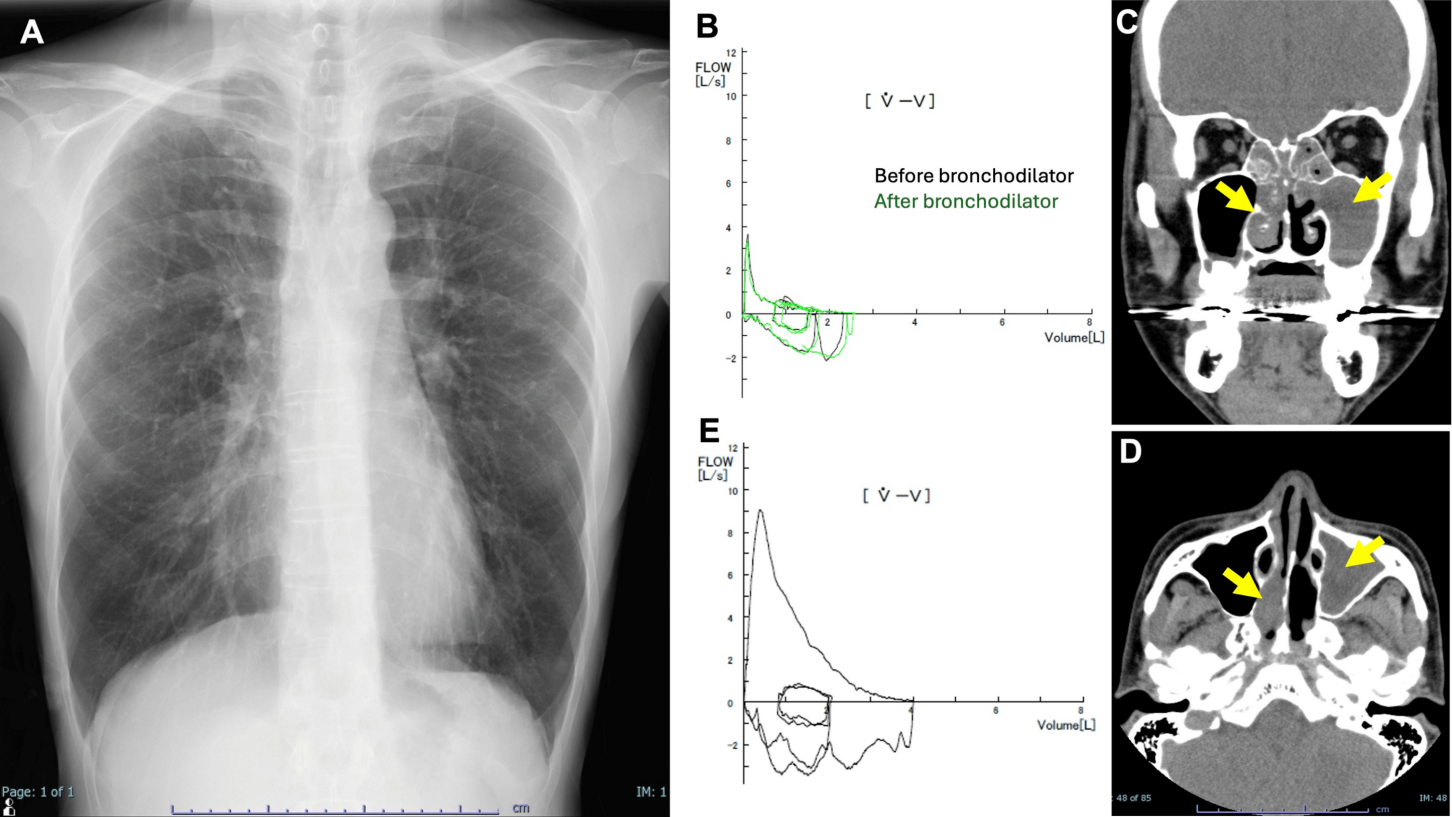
Radiographic and pulmonary function findings before and after treatment (A) Chest X-ray at initial presentation. (B) Flow-volume curve at initial presentation, demonstrating severe obstructive ventilatory impairment. The black line represents the flow-volume curve before bronchodilator administration, while the green line shows the curve after bronchodilator use. (C, D) CT of the paranasal sinuses demonstrated extensive soft tissue density areas filling the left maxillary sinus, extending to the ethmoid, sphenoid, and frontal sinuses (arrows). (E) Flow-volume curve eight months after initiation of tezepelumab treatment, indicating significant improvement in lung function. CT: computed tomography
